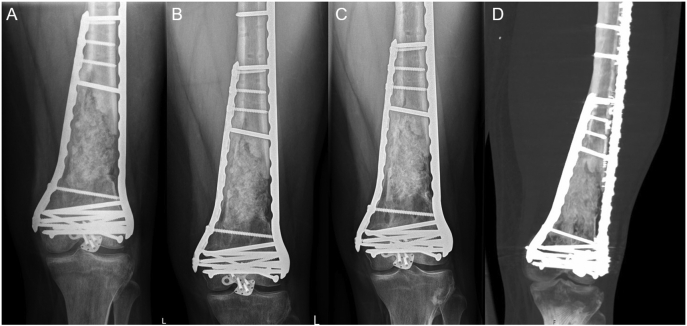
This figure shows the fracture consolidation over time. The radiograph at three months postoperative (A) shows both medial and lateral LCP-plates stabilizing the distal femur and an arrow-shaped plate for osteosynthesis of the patella. There are no signs of loosening or graft resorption. The interposed bone graft is partially integrated. At six months postoperatively (B), progredient consolidation and integration of the interposed bone graft can be stated. 12 months postoperatively, consolidation of the fracture with complete integration of the bone graft and cortical bone formation could be found on the radiographs (C) and computer tomographic imaging (D).
